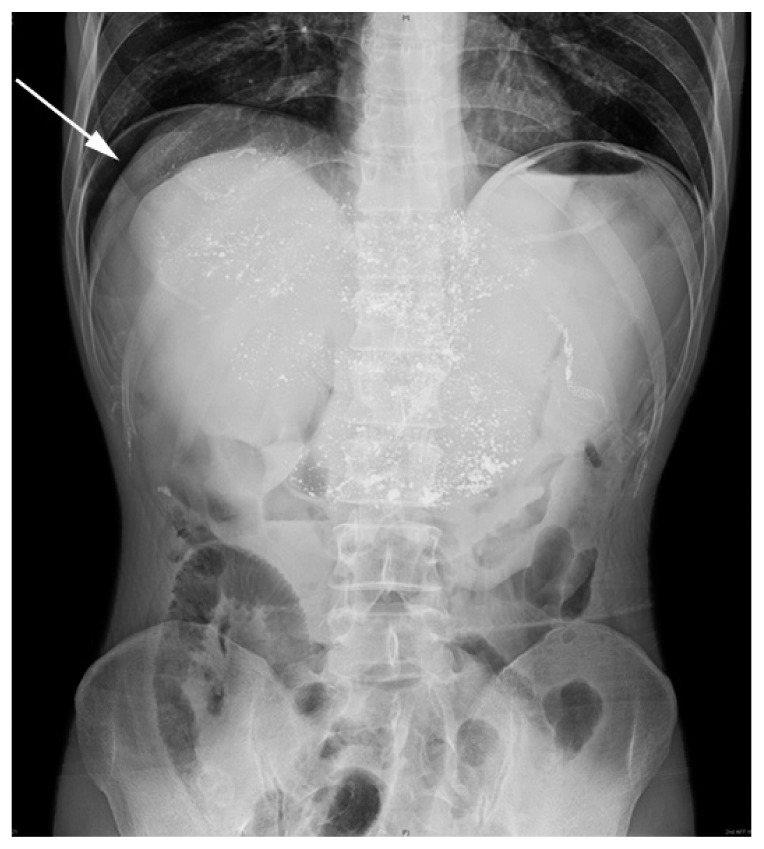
Plain X-ray of the abdomen revealed little free gas in the subphrenic spaces (arrow) in a 41-year old male patient with late anastomotic perforation following surgery for gastric neuroendocrine tumor.
